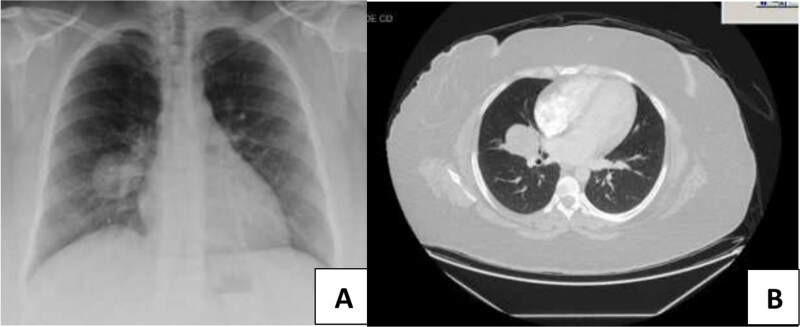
Radiological examination of the chest of the patient. (A) Chest X-ray revealed a demarcated nodule located in the middle right lobe. (B) Chest CT scan displayed a 4.1 cm nodule with no mediastinal lymph node enlargement.
